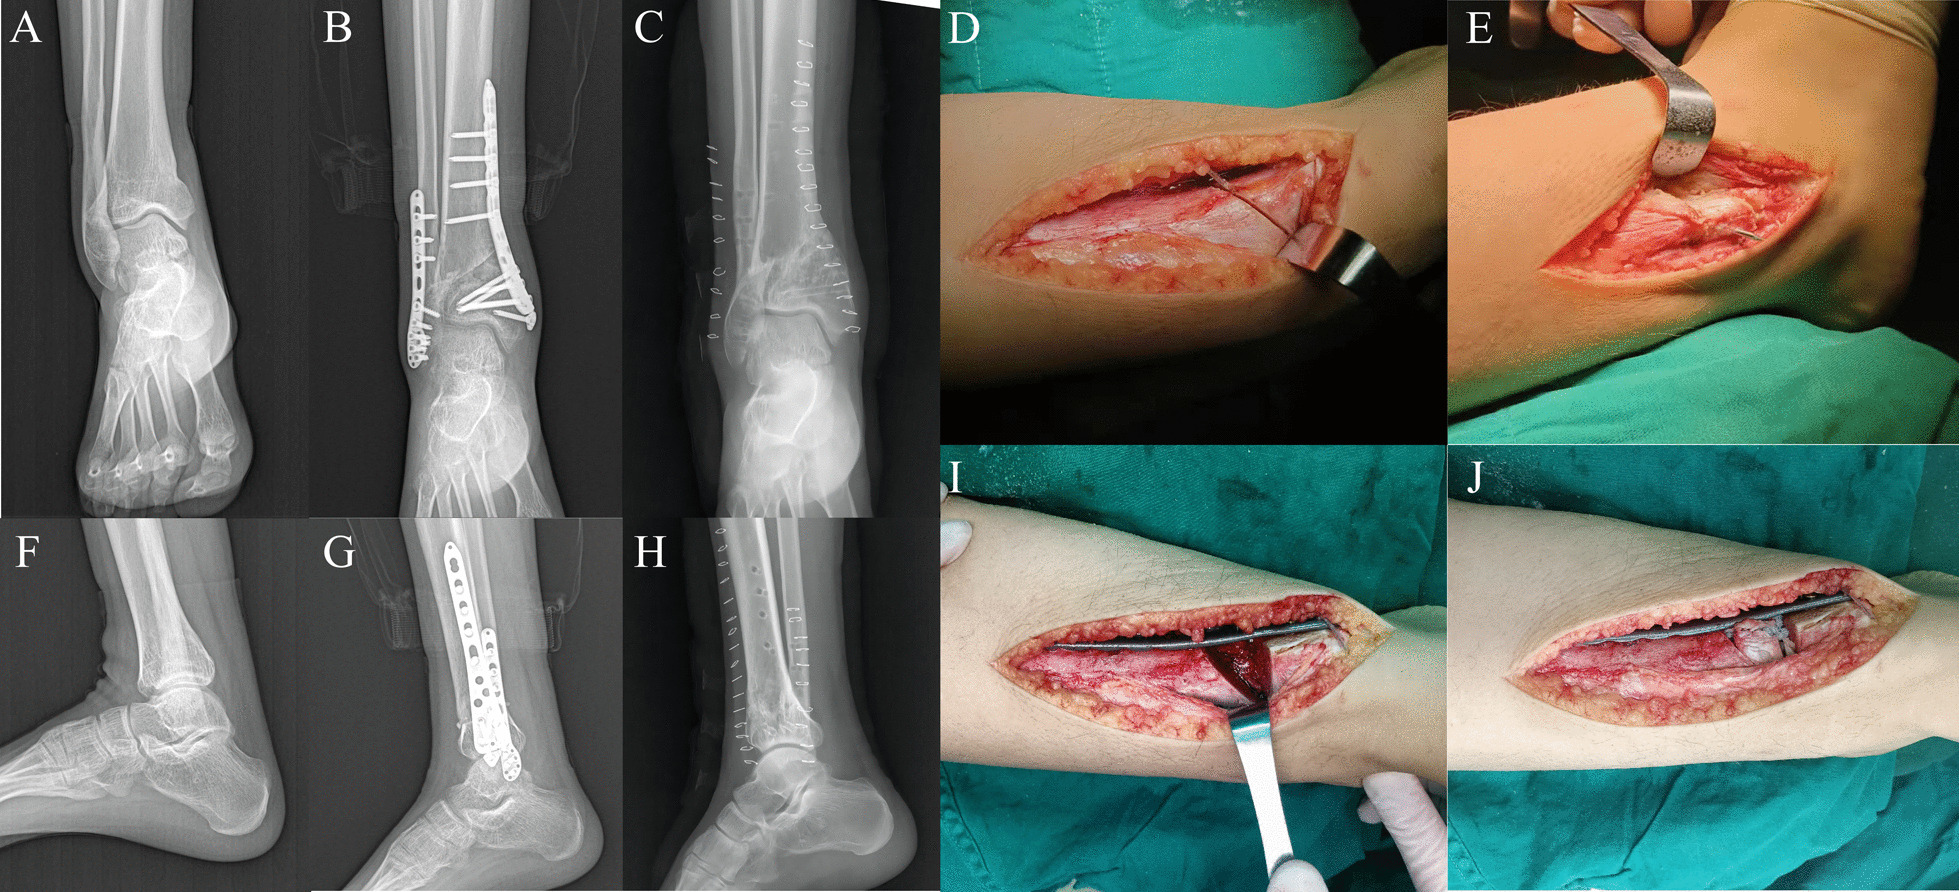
Radiographic follow-up and intraoperative photographs of a 16-year-old patient who underwent Supramalleolar osteotomy for treatment of traumatic ankle joint varus deformity. A is a preoperative anterior posterior radiograph, B is a 3-month postoperative anterior posterior radiograph, C is a 12-month postoperative anterior posterior radiograph, D is a medial ankle incision and osteotomy based on preoperative planning, E is a fibula osteotomy, F is a preoperative lateral radiograph, G is a 3-month postoperative lateral radiograph, H is a 12-month postoperative lateral radiograph, I is to spread the osteotomy surface and check that the distal tibia medial anatomic locking plate can be placed successfully, and J is the use of autogenous bone implantation at the osteotomy site followed by placement of a distal tibia medial anatomical locking plate for fixation
